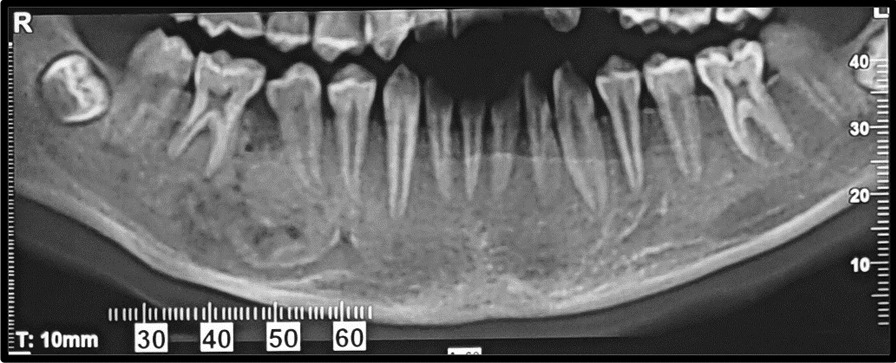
cone bean computed tomography image (panoramic reconstruction) revealed an ill-defined mixed lesion in the right side of the mandibular body extending from mesial aspect of right first premolar to periapical region of right first molar. Note the Inferior displacement of inferior alveolar canal and root divergence of 2nd premolar and 1st molar
